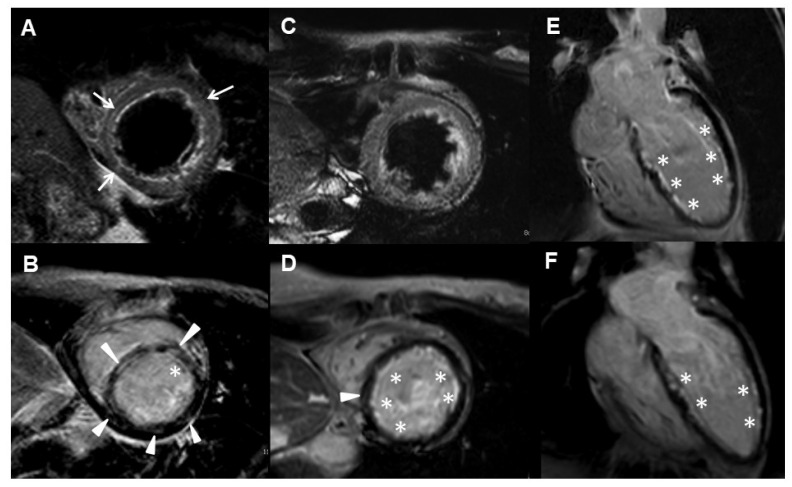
Serial cMRI images in case no. 3 before and after RTX therapy. (A,B) Pre-RTX treatment cMRI images revealed mid-wall edema in short-axis T2-weighted images (A, white arrows) and post-gadolinium delayed enhancement images (B, white arrowheads). Regional endocarditis in the anterior wall at LV mid-cavity (B, asterisk). (C–E) After induction therapy, follow-up images showed resolved myocardial edema (C), some mid-wall fibrosis (D, white arrowheads) and interval worsening of endocarditis (D,E, asterisks) seen from short-axis and 4-chambered sections. (F) The endocardial change revealed improvement after two courses of maintenance therapy (F, asterisks).
